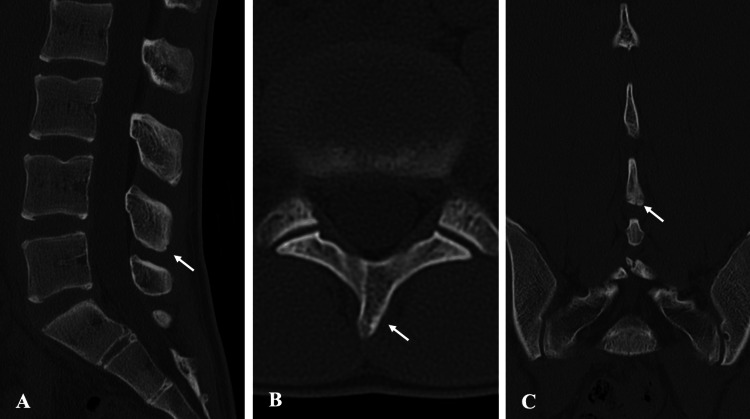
Pre-treatment CT findings in Case 1. CT shows no evidence of fracture at the corresponding site: (A) sagittal view, (B) axial view, and (C) coronal view. CT, computed tomography
